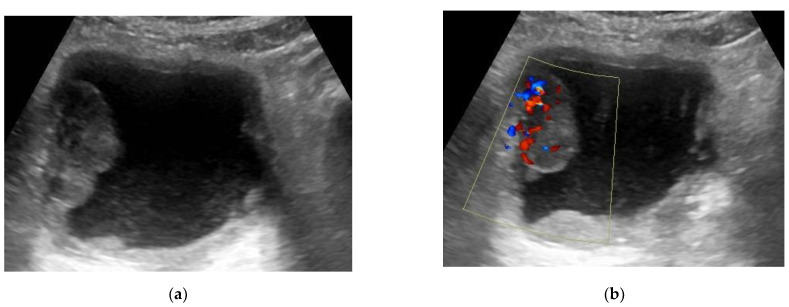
(a) Transverse US (ultrasound) image of the bladder showing a large well-defined echogenic mass (4 × 2 × 3.7 cm) at the right lateral bladder wall. (b) Color Doppler ultrasound detected the presence of vascularity within this mass. (This mass was pathologically proven to be urothelial carcinoma, stage T2 N0).
